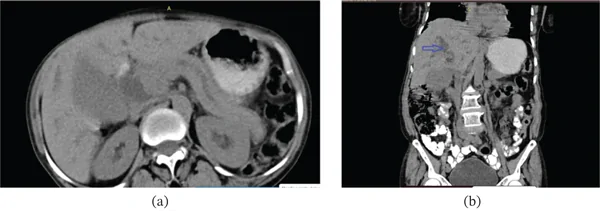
(A) A triphasic CT scan of a hypodense lesion on the head of the pancreas, with bile duct dilation. (B) A coronal view with evidence of hepatomegaly, hepatic hypodense infiltrates (see arrow), and a mass at the head of the pancreas.
